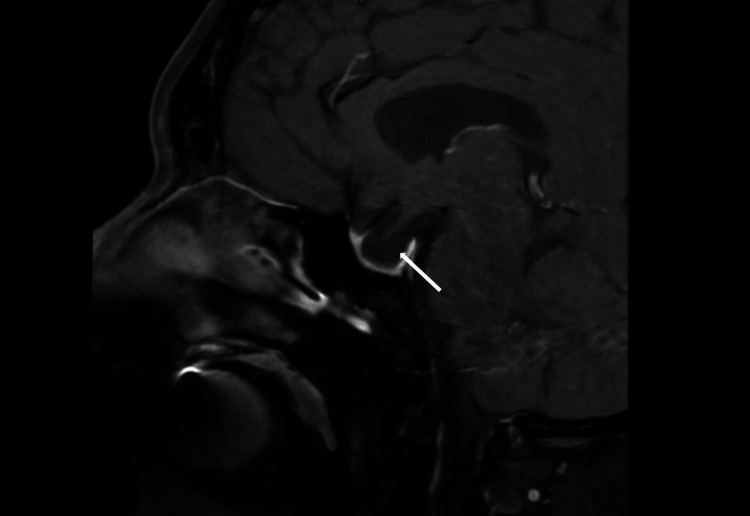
Sagittal T1-weighted MRI of the brain showing enlarged empty sella turcica (white arrow).
